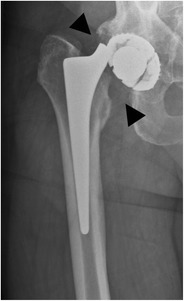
AP radiograph. Cementless total hip arthroplasty. Proximal prosthetic/metallic fracture through the neck of the femoral implant (*arrowheads*)
